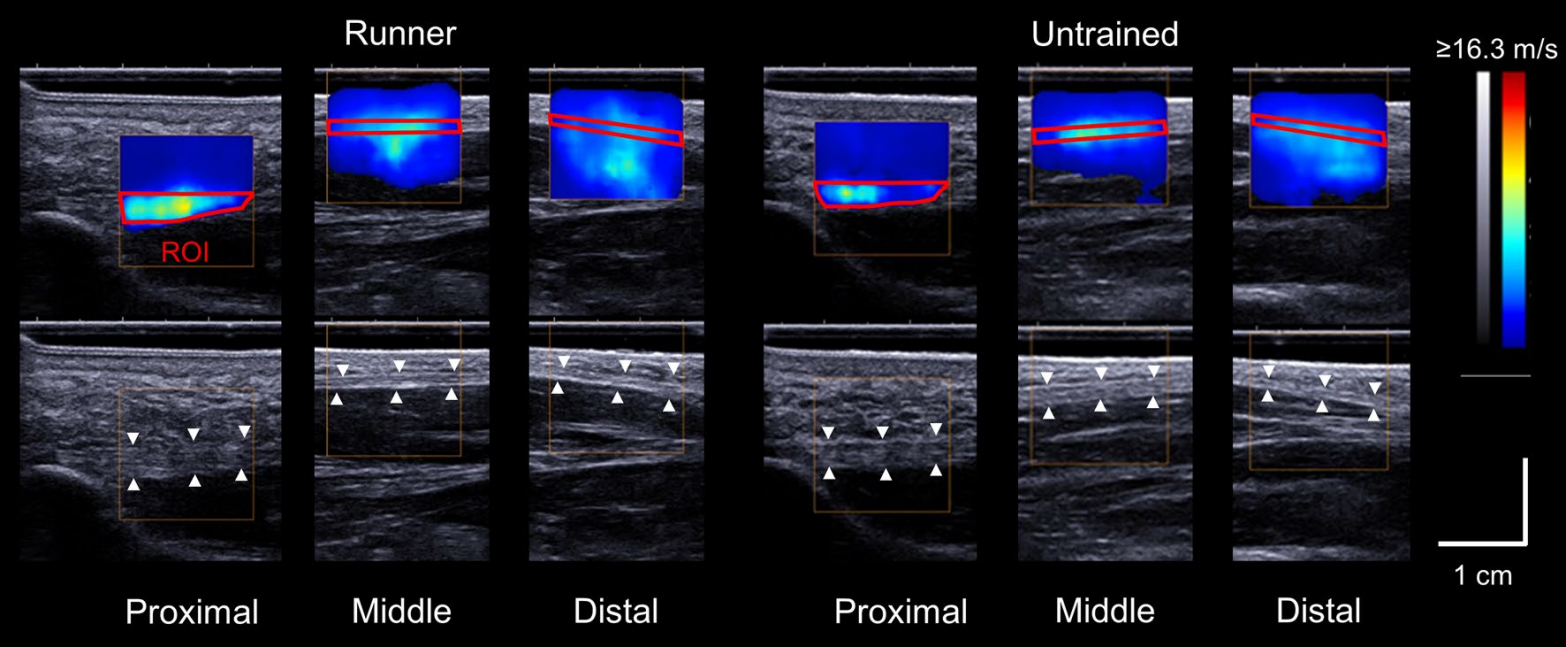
Representative shear wave and ultrasound B-mode images of the plantar fascia at the proximal, middle, and distal sites in a runner and an untrained participant. The region of interest (ROI) is bounded in red.
